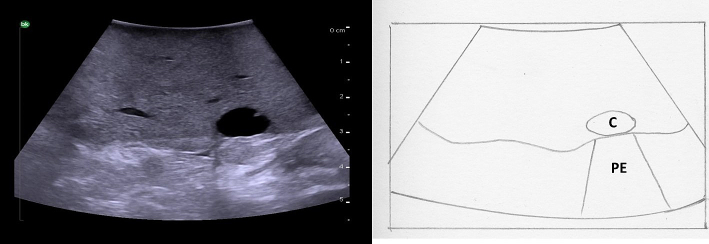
Intraoperative ultrasound appearance of an anechoic liver cyst with posterior enhancement. C cyst, PE posterior enhancement.
